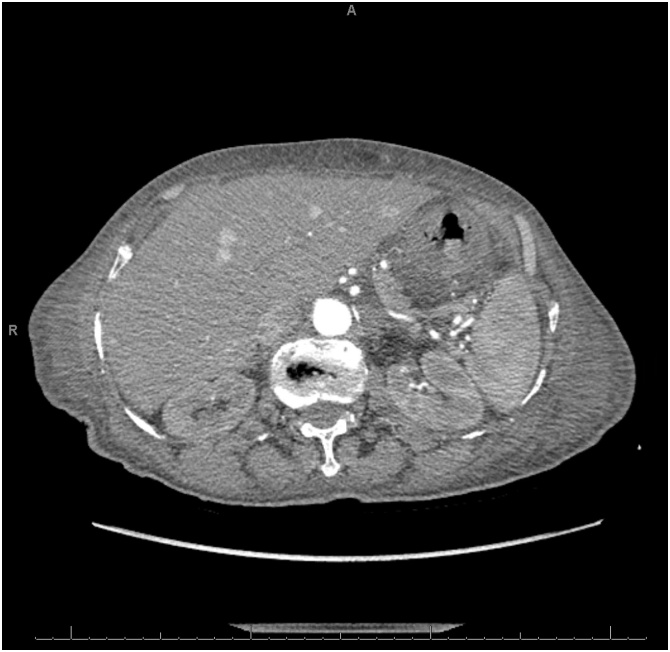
Computed tomography scan of the abdomen with contrast three days after the first scan showing resolution of the gastric pneumatosis and portal venous gas.
